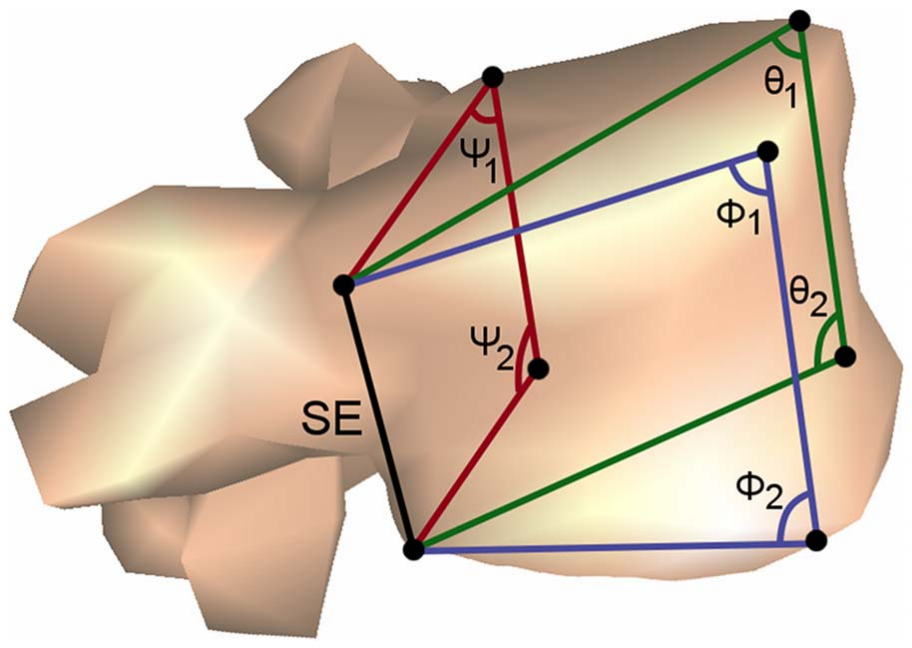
Selected landmarks and measured angles on each thoracic and lumbar vertebrae. Points represent the eight vertebral body corners as seen on the radiographs. The spatial angles were measured with respect to the smallest edge (SE) representing the frontal, sagittal and diagonal planes wedging. Ψ, φ and θ represent respectively the postero-frontal, sagittal and diagonal angles with 1 and 2 indicating the superior and inferior angles. Each superior and inferior angles of a plane were summated to characterize vertebral wedging.
